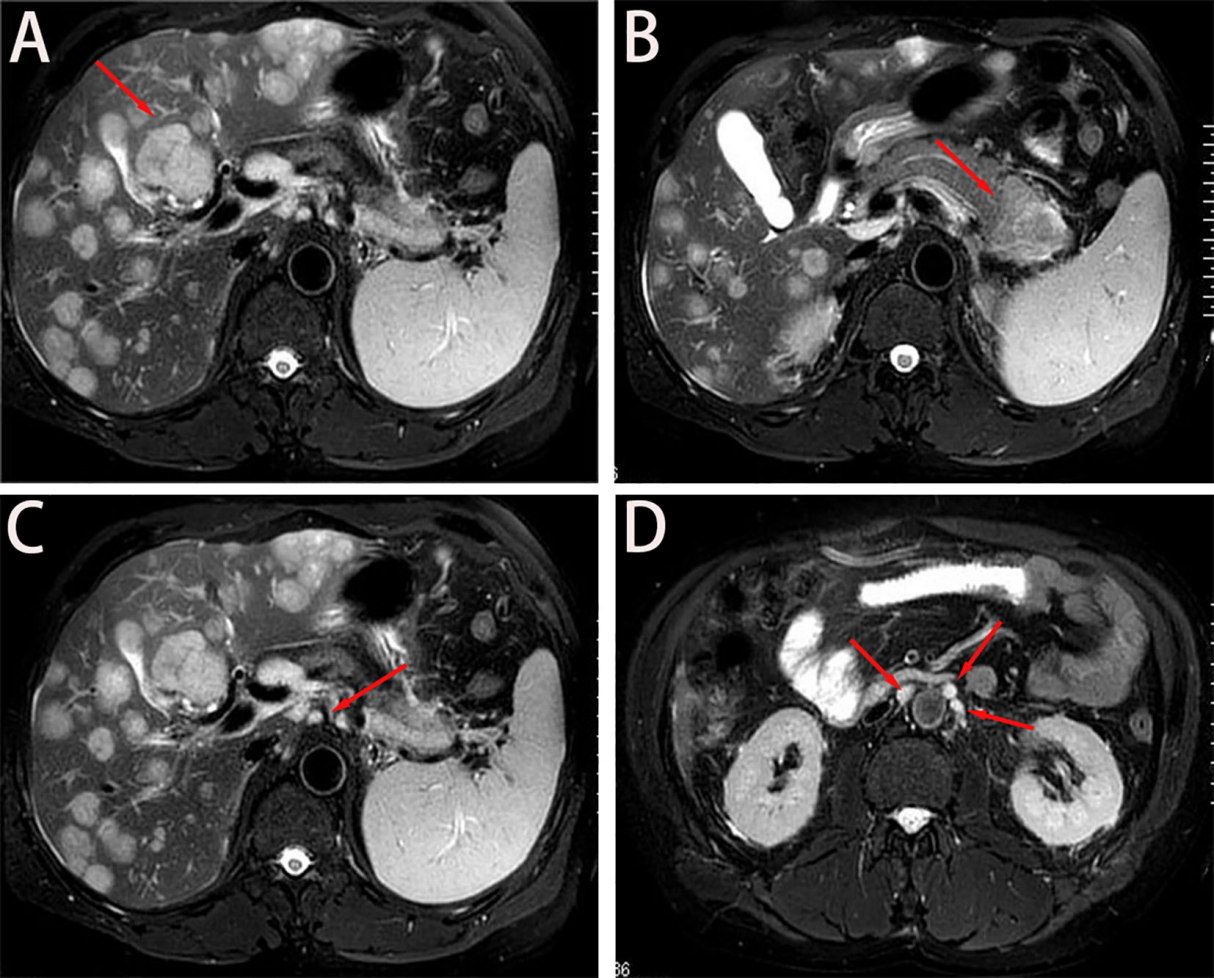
Baseline imaging findings of metastatic pancreatic adenocarcinoma. (A, B) Axial T2- weighted MRI demonstrates: (A) Diffuse hepatic metastases (arrows) with characteristic hyperintense signal; (B) A 4.7 × 3.7 cm² heterogeneously enhancing primary mass (asterisk) in the pancreatic tail. (C) Coronal view reveals metastatic involvement of the hepatic hilar lymph nodes (arrowheads), showing irregular margins and diffusion restriction. (D) Sagittal reconstruction identifies multiple enlarged retroperitoneal lymph nodes (circles) along the para-aortic region, consistent with metastatic spread.
